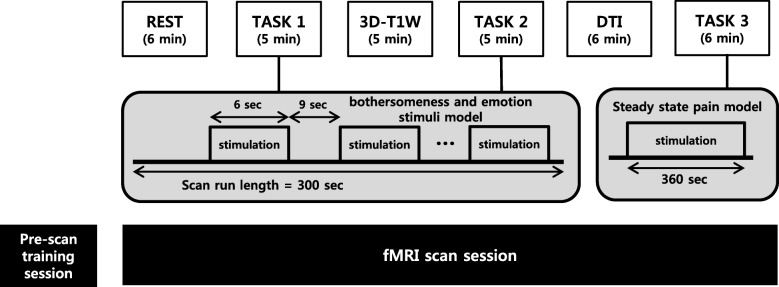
Functional magnetic resonance imaging experiment paradigm (*REST* steady-state resting run; *TASK 1/2* block design bothersomeness and emotion stimulus run; *3D-T1W* structural image acquisition using 3-dimensional T1-weighted turbo field echo; *DTI* diffusion tensor imaging; *TASK 3* low-back-extension pain-model steady-state run)
